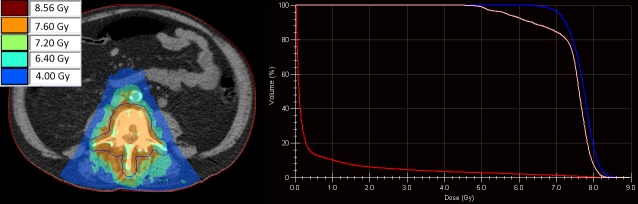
Dose distribution of the pre-treatment plan generated on the pre-treatment computed tomography (CT, left) with the corresponding dose volume histogram (right). Visible are the planning target volume (PTV, blue), spinal cord (pink), and body (red).
